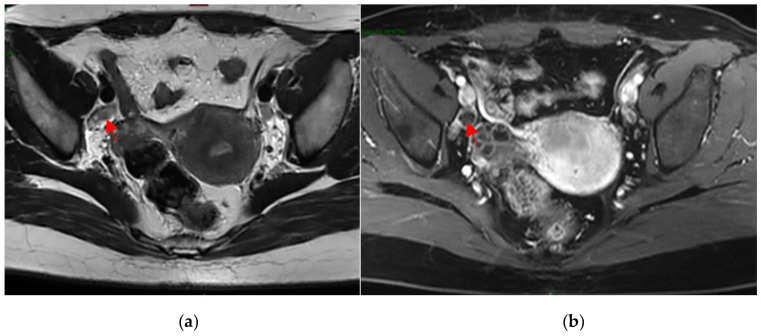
The right external lliac lymph node (arrow) of a 40-year-old EC patient. T2-weighted MRI (a) and contrast-enhanced T1-weighted MRI (b).
